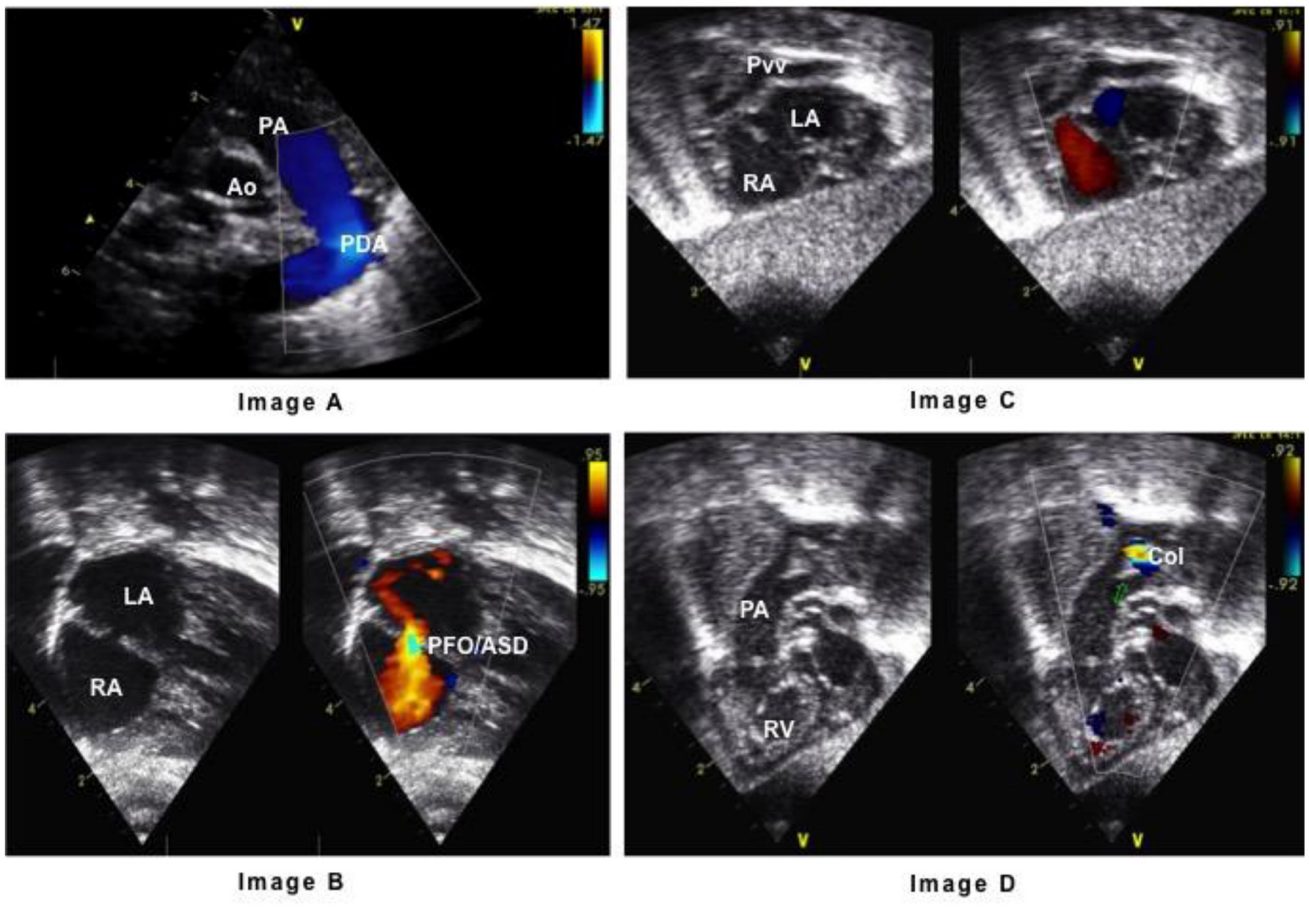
Echocardiography parasternal short axis view in systemic duct-dependent circulation with right-to-let shunt across the patent ductus arteriosus (Image A). Echocardiography subcostal view in transposition of the great arteries (TGA) with left-to-right interatrial mixing between the pulmonary and systemic circulation (Image B). Echocardiography subcostal view in total anomalous pulmonary venous return (TAPVR) with right-to-left interatrial mixing of the pulmonary and systemic circulation (Image C). The level of obstruction of pulmonary venous collector is usually at the branch pulmonary arteries (arrow, Image D). Ao, aorte; ASD, atrial septal defect; Col, pulmonary venous collector; LA, left atrium; PA, pulmonary artery; PFO, patent foramen ovale; PDA, patent ductus arteriosus; Pvv, pulmonary veins; RA, right atrium.
